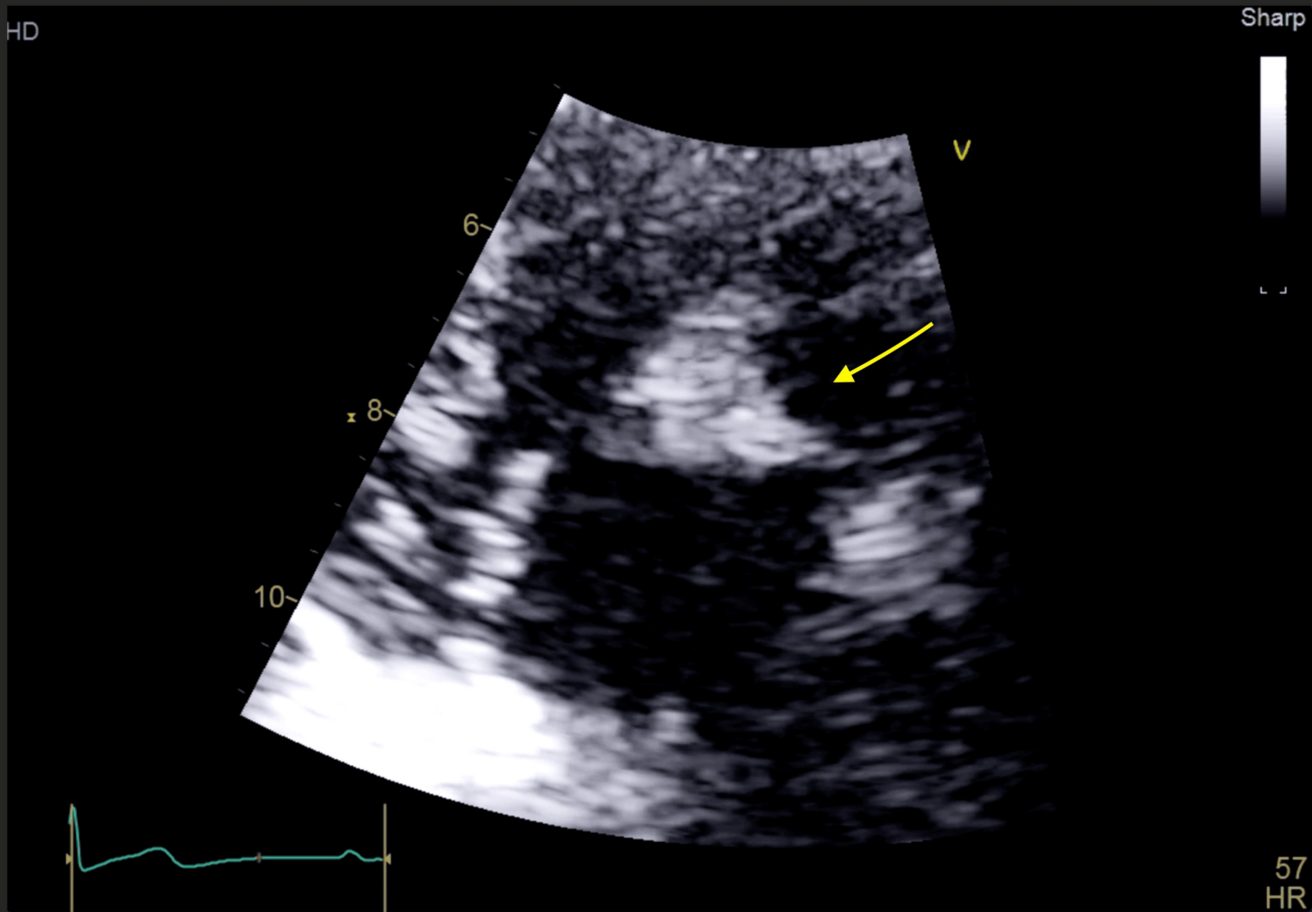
Transthoracic echocardiogram (parasternal long-axis view) demonstrating a large tricuspid mass. The arrow annotation demonstrates a large tricuspid mass within the right ventricular cavity.
